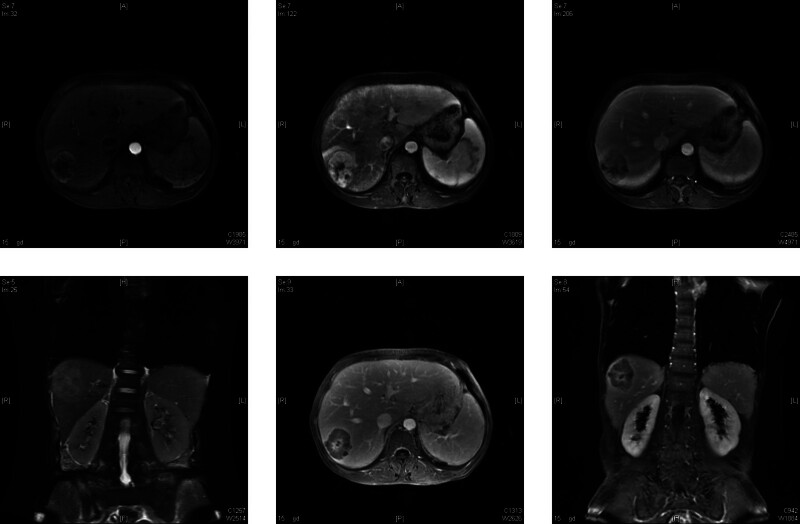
An abnormal mass was identified in the right lobe of the liver during an abdominal MRI scan with plain and dynamic enhancement.
